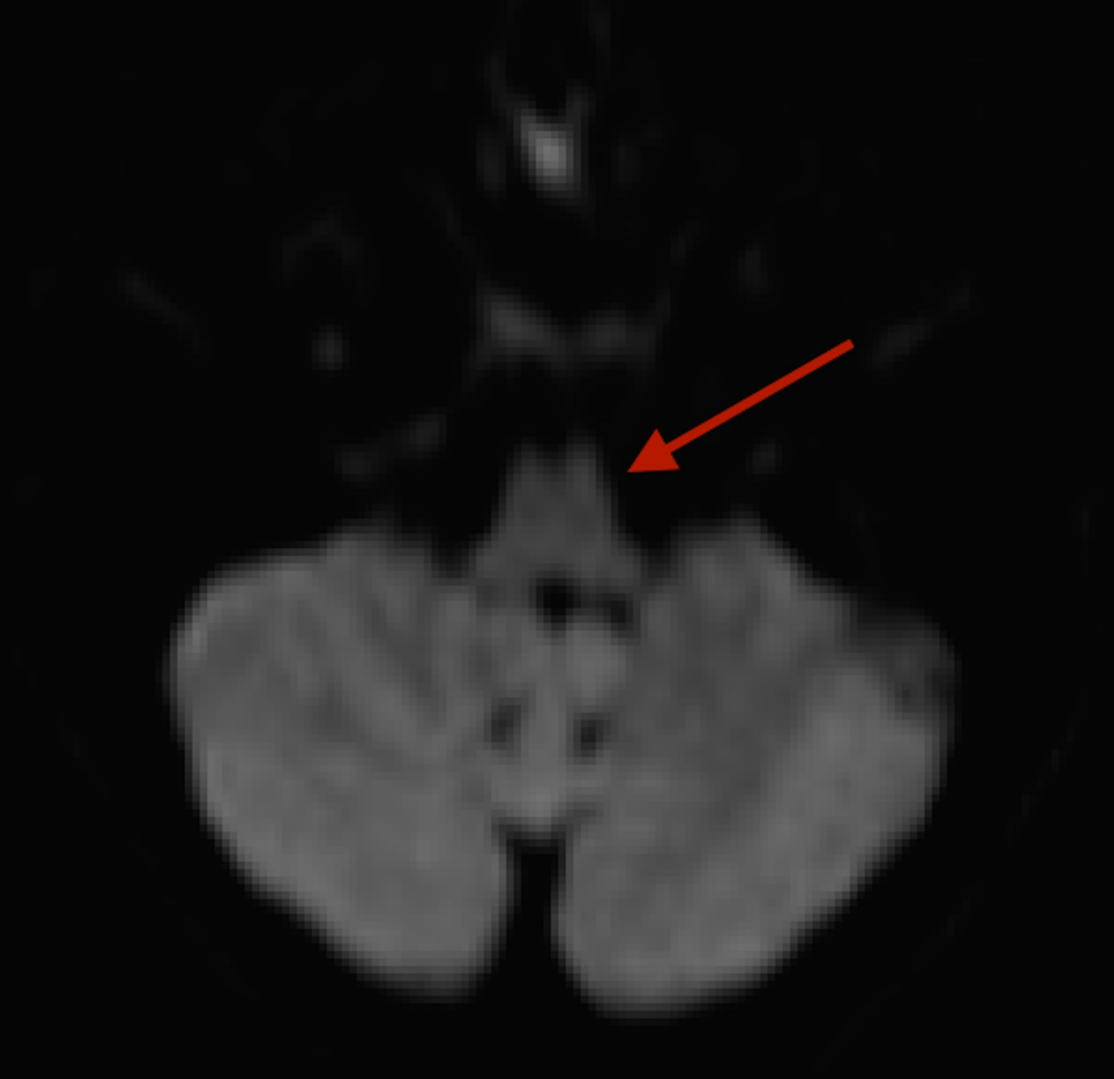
MRI Diffusion Weighted Imaging (DWI). No evidence of ischemic infarct of lower medulla (red arrow) MRI: Magnetic Resonance Imaging
